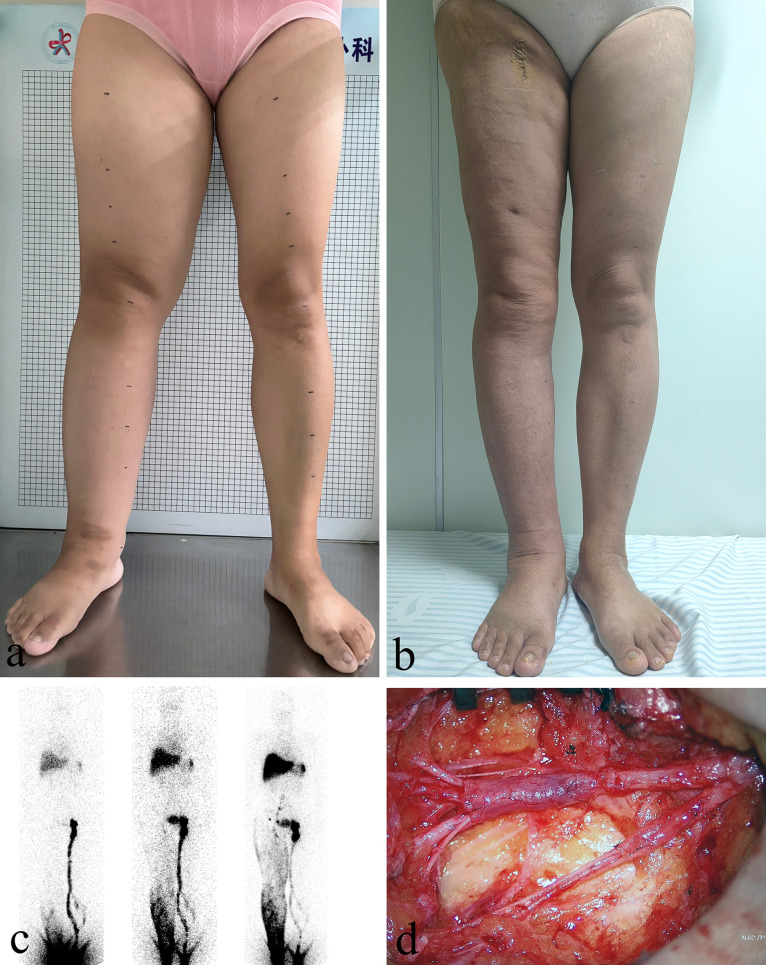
A 50-year-old woman with a history of cervical cancer surgery was diagnosed with right iliac vein compression 4 years ago and underwent stent implantation with no swelling relief. (A) Prior to the surgical procedure during the hospitalization stage, the patient’s right lower limb showed significant swelling that was classified as International Society of Lymphology Stage IIb. (B) After liposuction followed by lymphovenous anastomosis 3 months later, the swelling in the patient’s limb significantly improved. (C) Preoperative ^99^Tc^m^-DX lymphoscintigraphy in the anterior position at 10 min, one hour, and three hours post-injection demonstrated lymphatic return obstruction in the right lower limb. (D) The lymphatic vessels, the lateral femoral vein, and its branches were dissected at the inguinal level. After confirming good valve function, the lymphatic vessels were anastomosed to the lateral femoral vein and its branches.
